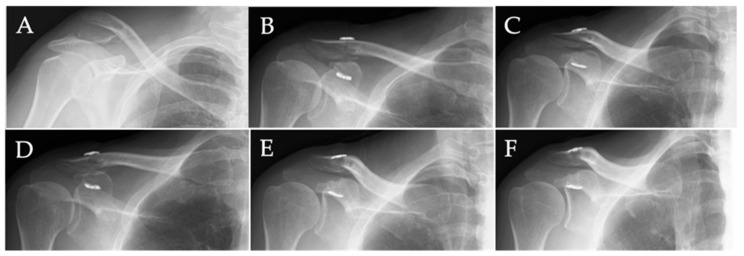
Representative X-rays before and after surgery. (A) Preoperative radiograph. (B) Postoperative radiograph 1 week after surgery. (C) Radiograph 3 months after surgery. (D) Radiograph 5 months after surgery showing complete bone union with subsidence of the clavicular button. (E) Radiograph 9 months after surgery showing no dislocation. (F) Radiograph 12 months after surgery showing no dislocation.
